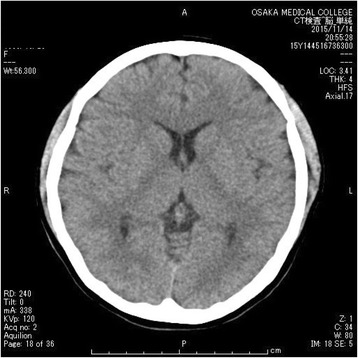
Head CT image with no remarkable findings
